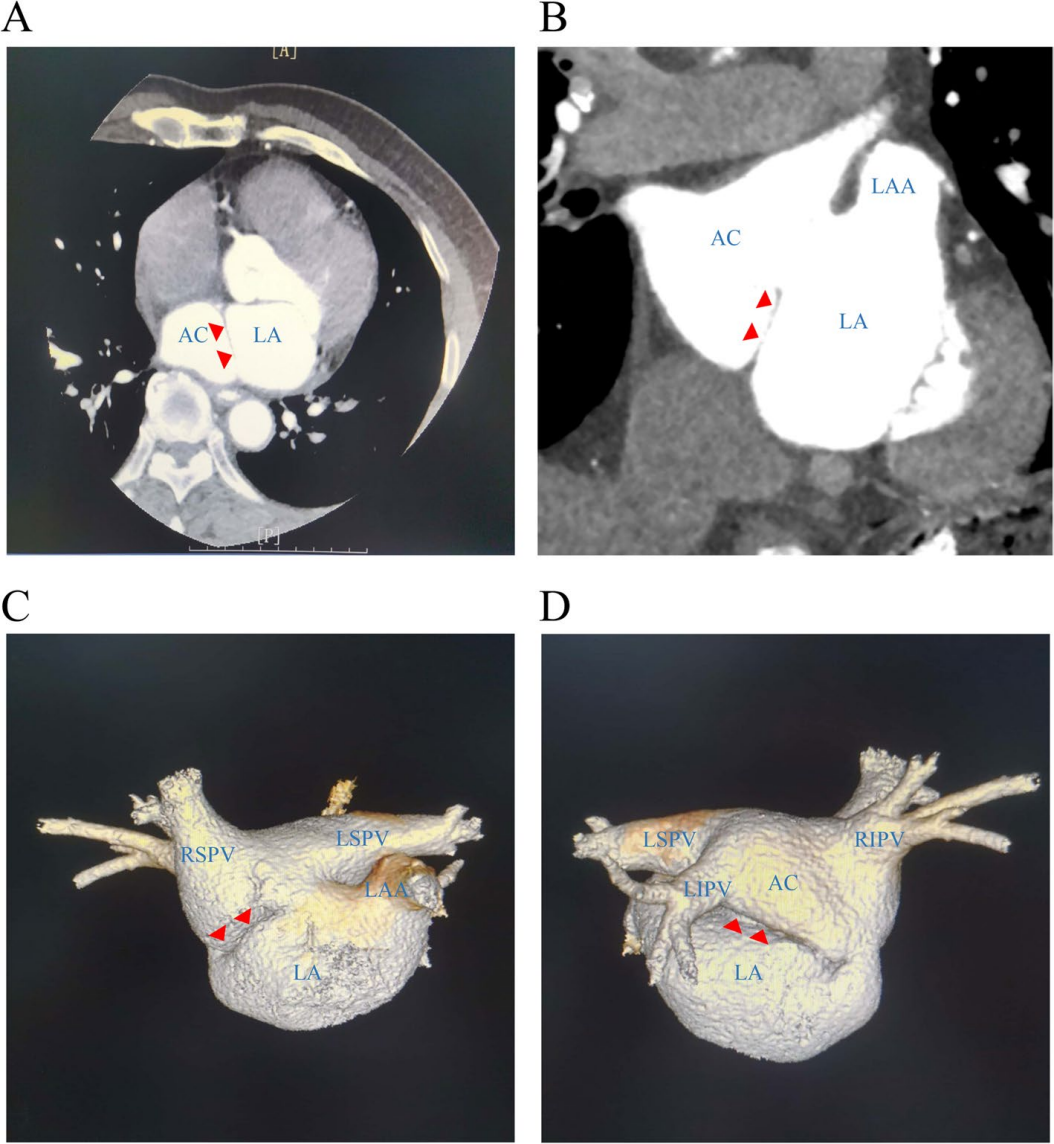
Images obtained using cardiac CTA. A Transverse view of the heart, red solid triangular arrowheads indicate the membrane dividing the left atrium into the accessory and true left atrial chambers. B Coronal view of the heart, red solid triangular arrowheads indicate the membrane dividing the left atrium into the accessory and true left atrial chambers. C, D Three-dimensional reconstruction of the left atrium by CTA. Red solid triangular arrowheads indicate the membrane. Four pulmonary veins drain into the accessory chamber and the true left atrial chamber contains the left atrial appendage (LAA) and mitral annulus. Mem: membrane; AC: accessory chamber; CTA: computed tomography angiography, LA: true left atrial chamber; LAA: left atrial appendage; LSPV: left superior pulmonary vein; LIPV: left inferior pulmonary vein; RSPV: right superior pulmonary vein; RIPV: right superior pulmonary vein
